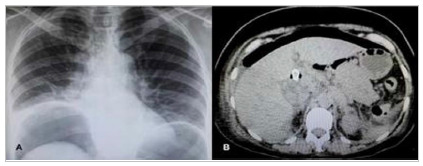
A) Chest X-ray showing pneumoperitoneum after EUS-CDT with metallic
